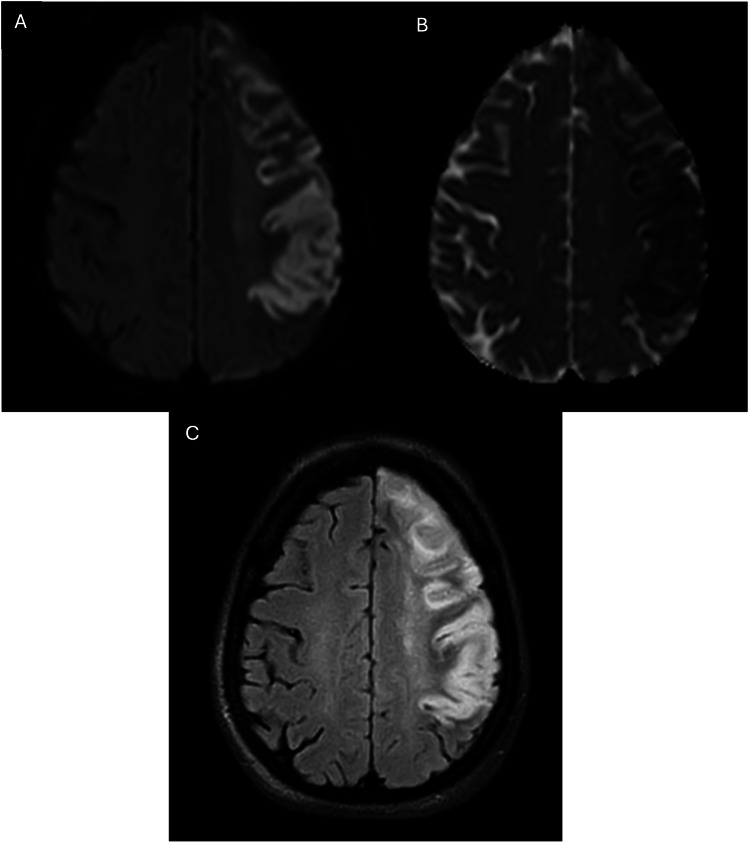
MRI of the brain showing: (A) Axial DWI image (b=1000), with its corresponding axial ADC image. (B) exhibiting expansion of the infarct to involve the left middle cerebral artery (MCA) region, affecting the left parietal lobe. Some evidence of pseudonormalization is already seen. (C) The corresponding axial FLAIR image shows an increased signal at the same region. DWI: Diffusion-weighted imaging; FLAIR: Fluid-attenuated inversion recovery; ADC: Apparent diffusion coefficient
